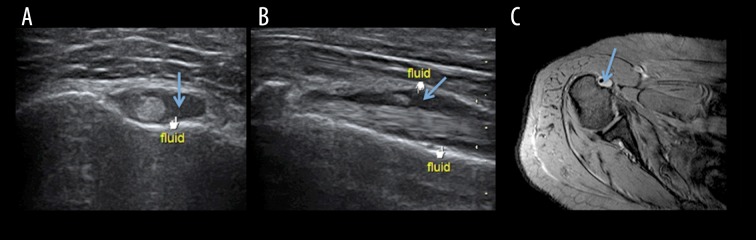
(A) USG, axial image showing a mild fluid accumulation in the bicipital groove (B) USG, longitudinal image showing a mild fluid accumulation along the biceps tendon (C) MRI, T2W axial image depicting a mild fluid accumulation in the bicipital groove.
